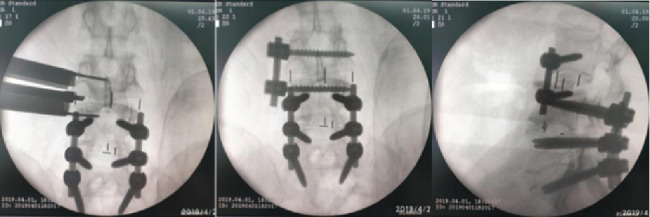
Radiographic images showed the surgical process of OLIF with lateral screw fixation.
